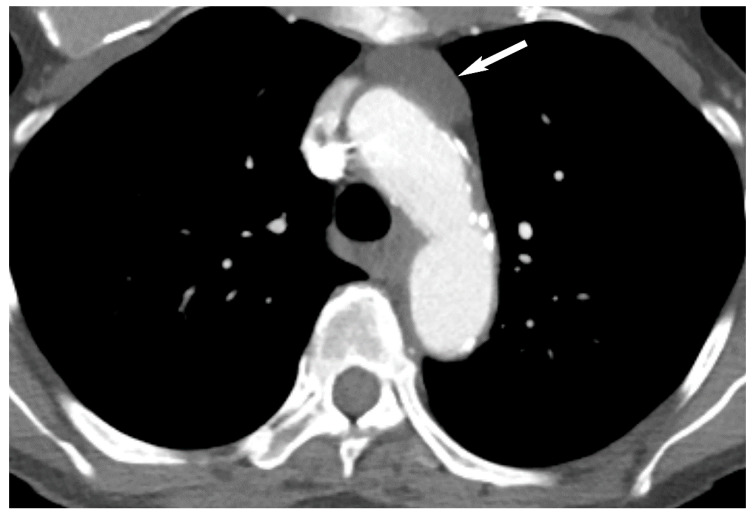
Thymic cyst. CT shows a fluid attenuating structure (15 Hounsfield units) in the prevascular mediastinum in the region of thymic bed (arrow).
